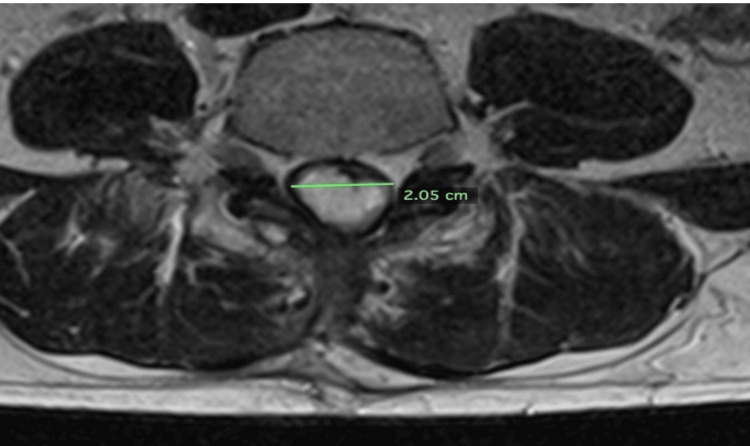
Axial image from an MRI L-spine with contrast dated November 16, 2022. The tumor measured 2.05 cm transversely.
